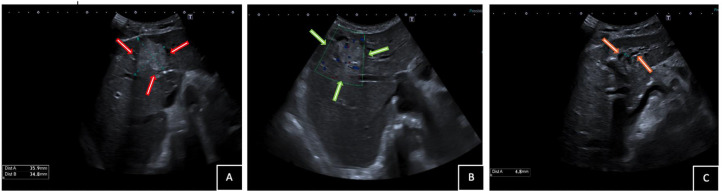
Abdominal ultrasound images show (A) a hyperechoic solid mass of 36 mm in the left hepatic lobe (red arrows) with (B) mild vascularization on Doppler (green arrows) causing (C) left intrahepatic ductal dilatation (orange arrows).
